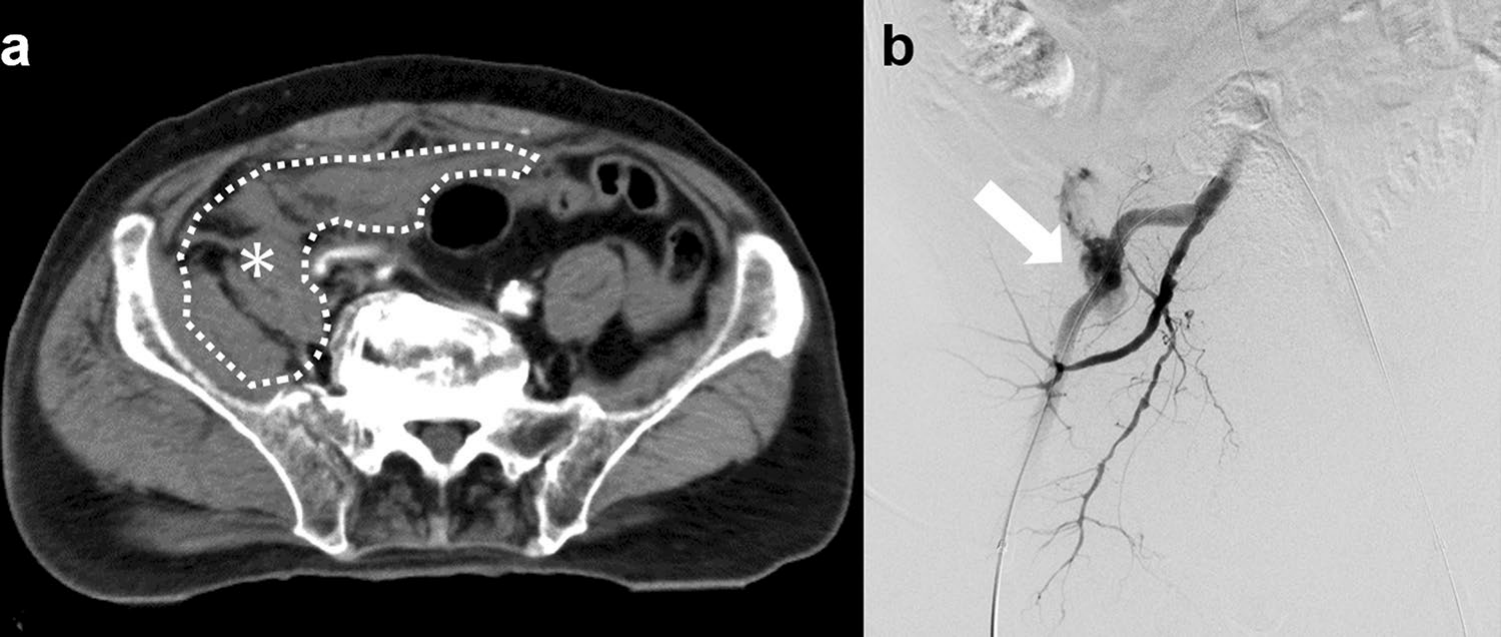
Pelvic contrast-enhanced CT images obtained during complete REBOA. a Delayed-phase (second phase) contrast-enhanced CT image shows a massive hematoma (asterisk) without extravasation of the contrast material. b Digital subtraction angiography of the right internal iliac artery obtained during REBOA balloon deflation shows massive extravasation from the iliolumbar artery, which represents “hidden” bleeding on contrast-enhanced CT during complete REBOA (arrow). We embolized the iliolumbar artery with NBCA. *CT* computed tomography; *REBOA* resuscitative endovascular balloon occlusion of the aorta; *NBCA*
